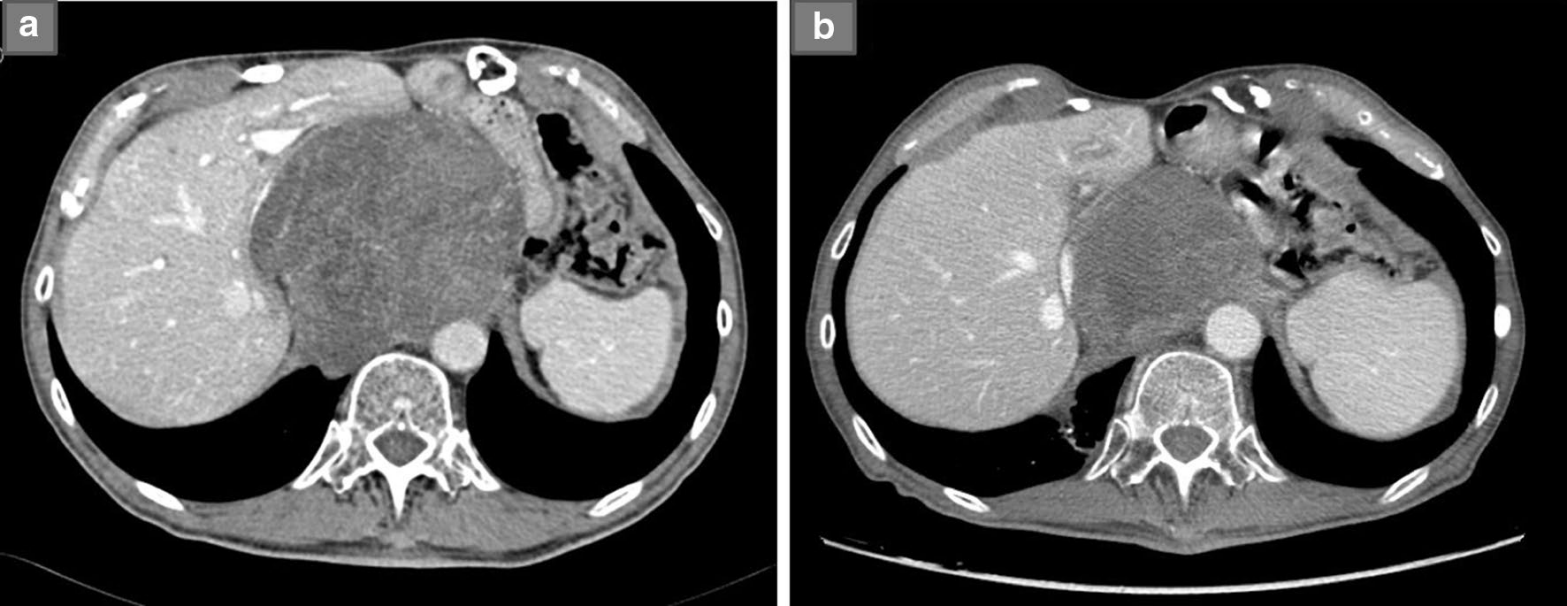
a CT scan documenting a voluminous GIST metastasis compressing the vena cava (diameter 130 × 110 mm). b CT scan performed 4 months after radiotherapy showing significant tumor shrinkage
